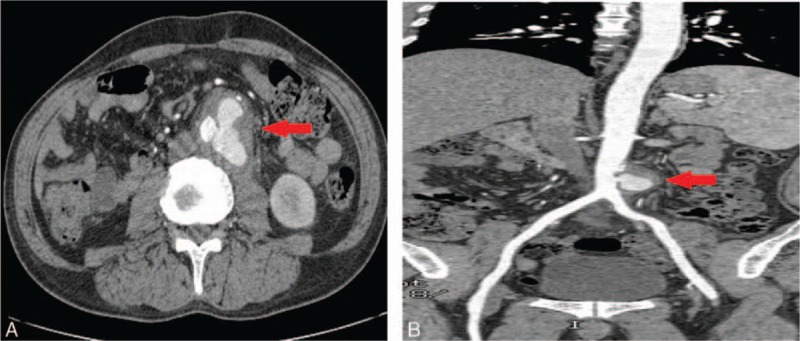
An abdominal aortic pseudoaneurysm observed on 3D-CTA (A and B, arrows). 3D-CTA = three-dimensional computed tomography angiography.
